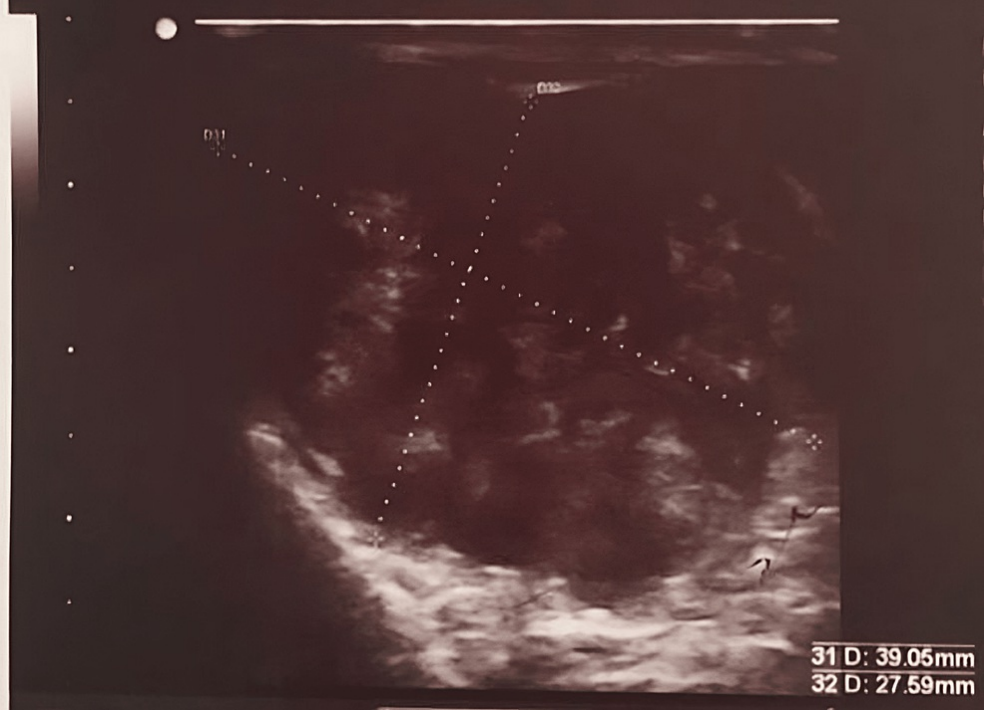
Scrotal ultrasonography (US) showed a well-limited heterogeneous hypoechoic intratesticular mass measuring 3.9 x 2.7 cm on the left testis.
